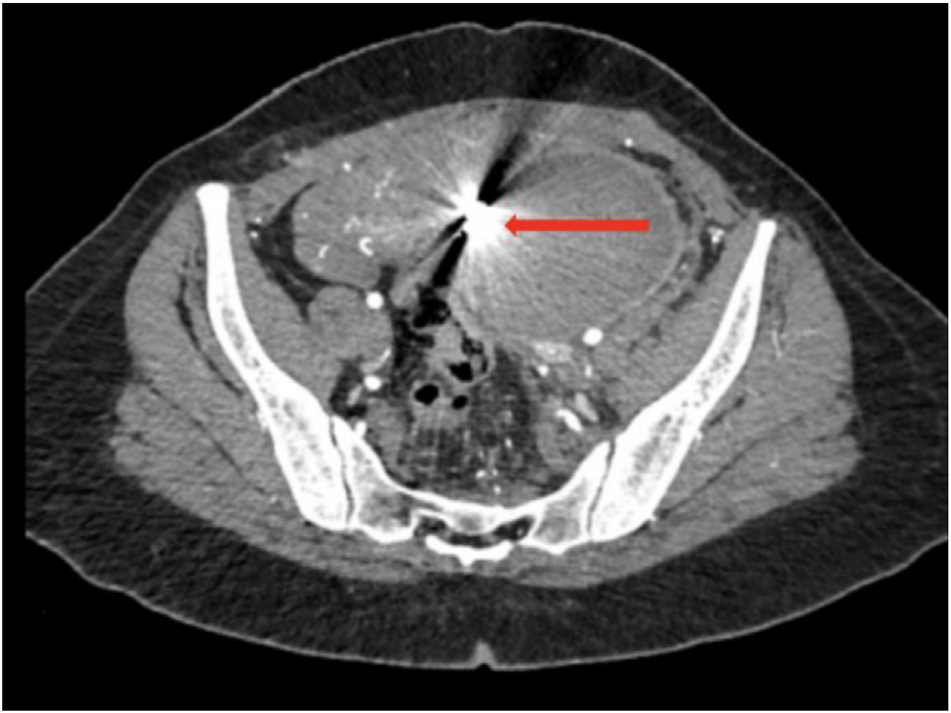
Axial arterial phase CT image showing embolization coils in place (red arrow) with complete disappearance of the previously noted pseudoaneurysm.
